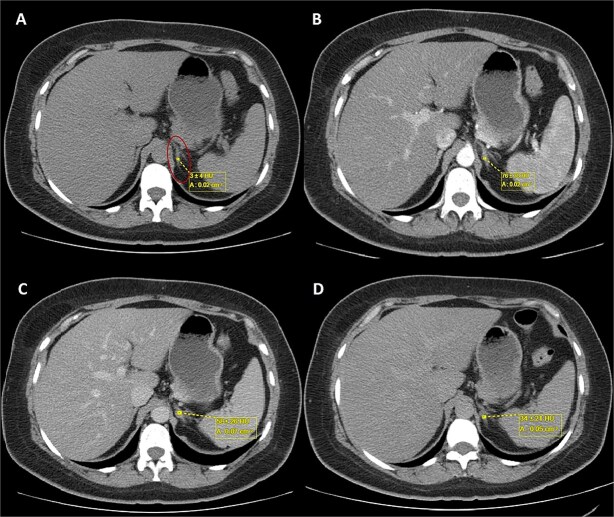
Triphasic abdominal CT scan with left adrenal adenoma (ellipse). (A) Simple phase, (B) arterial phase, (C) venous phase, (D) late venous phase.
